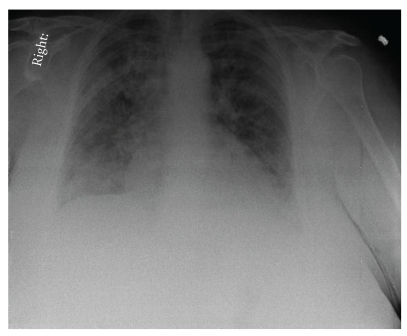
Chest radiograph showed acute bilateral pulmonary infiltration, more confluent in the areas of the right upper and bilateral lower lobes.
